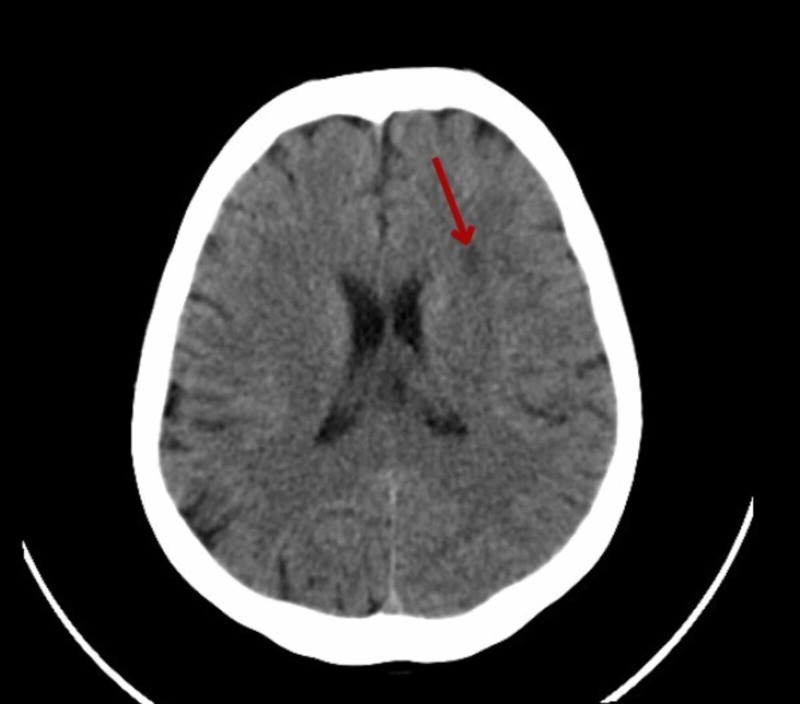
Computed tomography (CT) of the brain showing an ill-defined hypodense area in the deep white matter of the left frontal lobe
